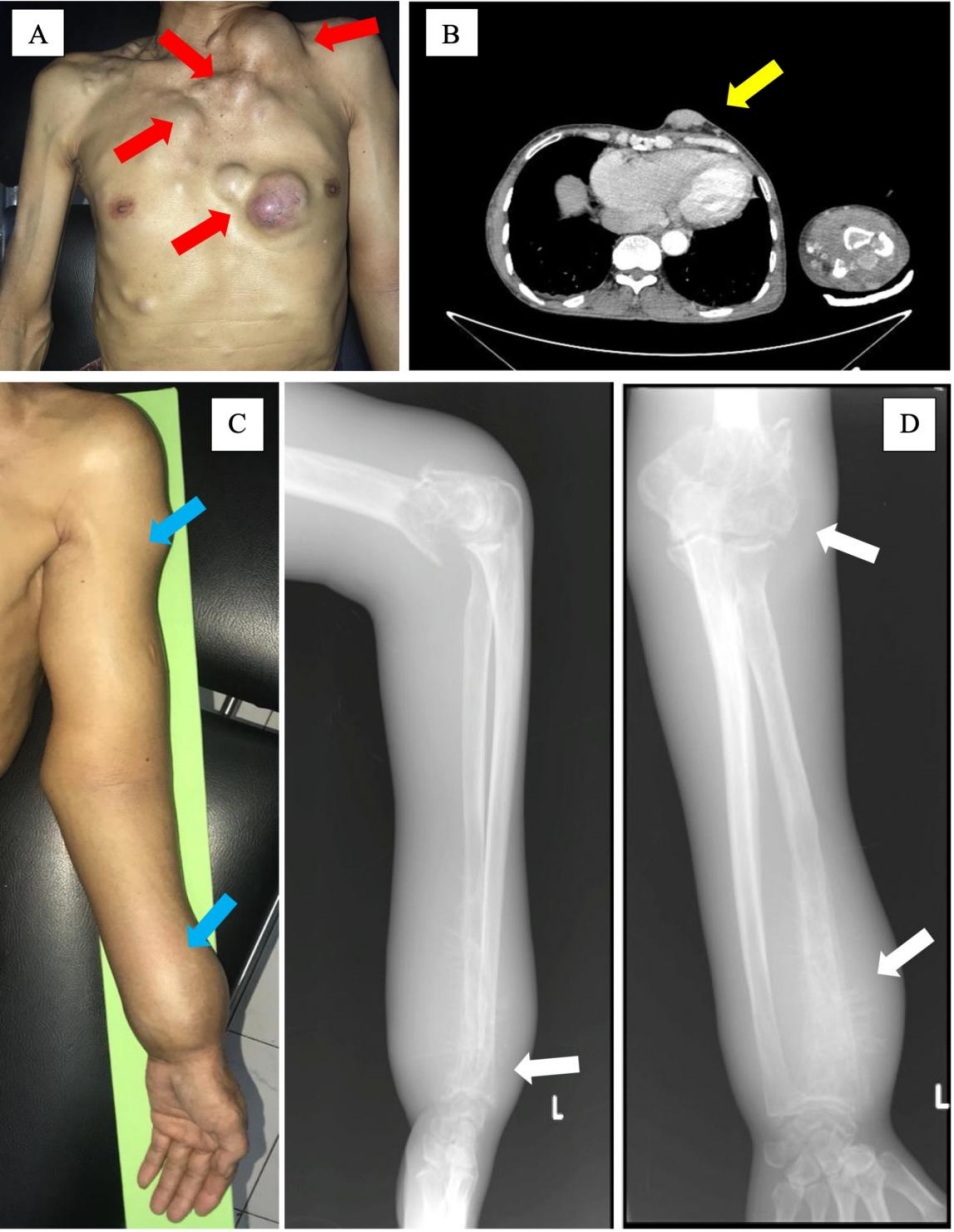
(A) Photograph of the patient showing multiple nodules in the chest (indicated by red arrows). (B) Multislice computed tomography (MSCT) image showing the subcutaneous soft tissue mass (indicated by the yellow arrow). (C) Photograph of the arm showing deformities caused by multiple fractures (blue arrows). (D) X-ray images confirm the fractures (white arrows).
